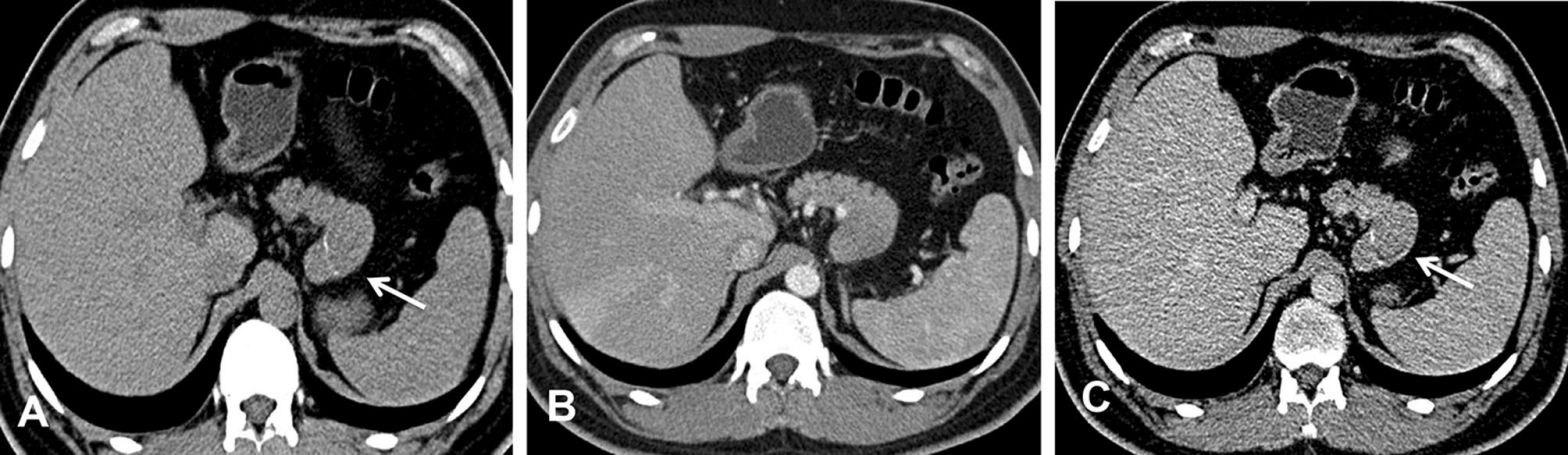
CT images of a 36-year-old man with surgically confirmed PB. (A) Nonenhanced CT scan revealed an exogenous mass with calcification in the tail of the pancreas (arrow). (B) The tumor exhibits an exophytic growth pattern, similar to branches growing outward from a tree trunk. In the AP, the tumor showed uniform enhancement, but the degree of enhancement was lower than that of the normal pancreatic parenchyma. (C) In the VP, the tumor presented as a well-defined mass with enhanced encapsulation (arrow) that had slightly less enhancement than the normal pancreatic parenchyma.
